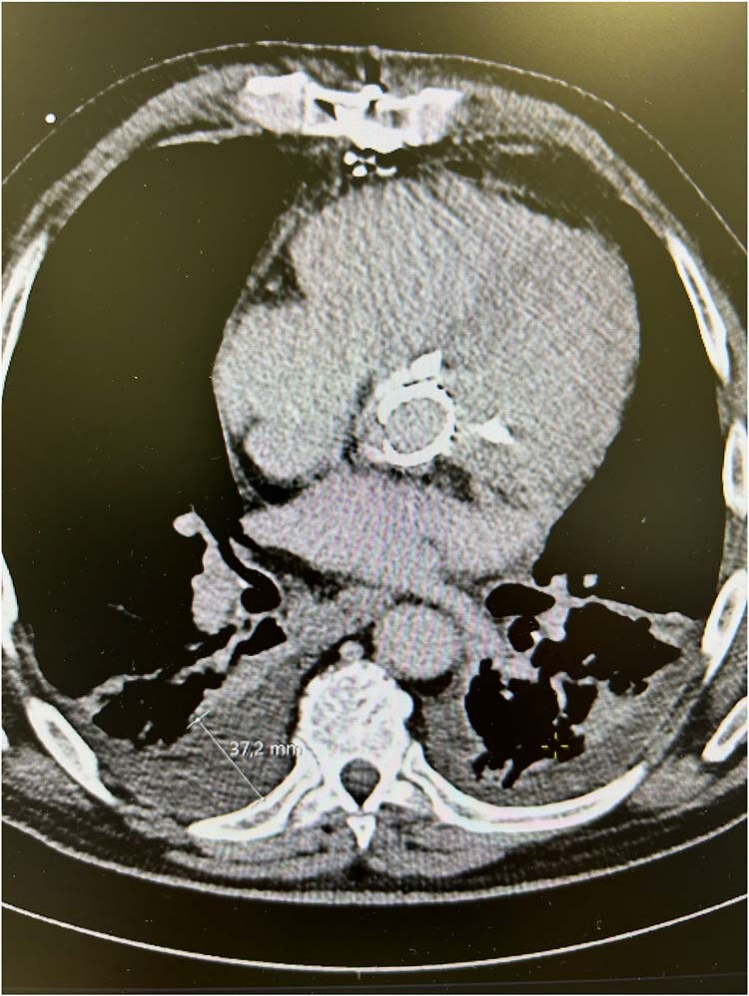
Preoperative computed tomography scans showing calcified aortic valve bioprosthesis in the aortic prosthesis together with pleural effusions.
